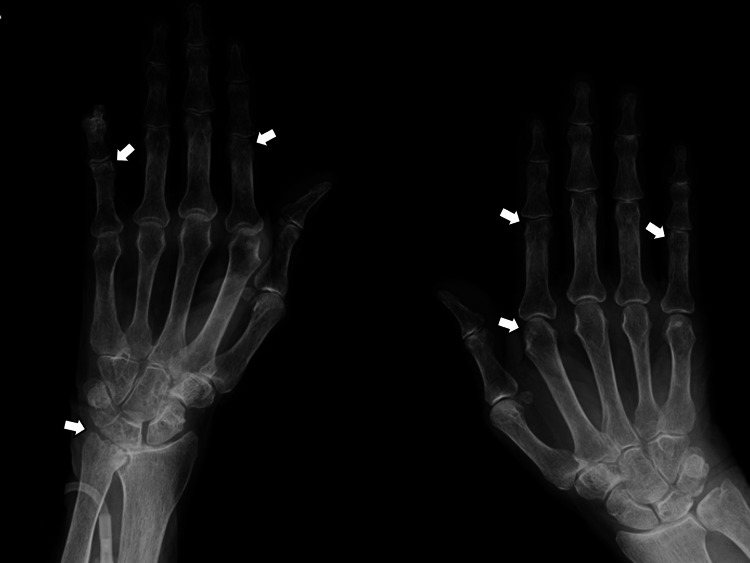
Radiography of the hands revealing bony erosions, consistent with rheumatoid arthritis (white arrows)
